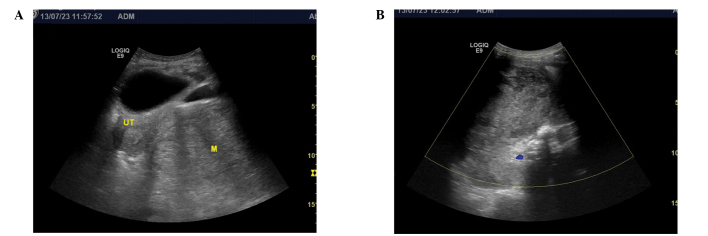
Abdominal and pelvic ultrasound. (A and B) Solid heterogeneous retroperitoneal masses are evident, with multiple scattered areas of increased echogenicity and dense posterior shadowing suggestive of foci of calcification.
